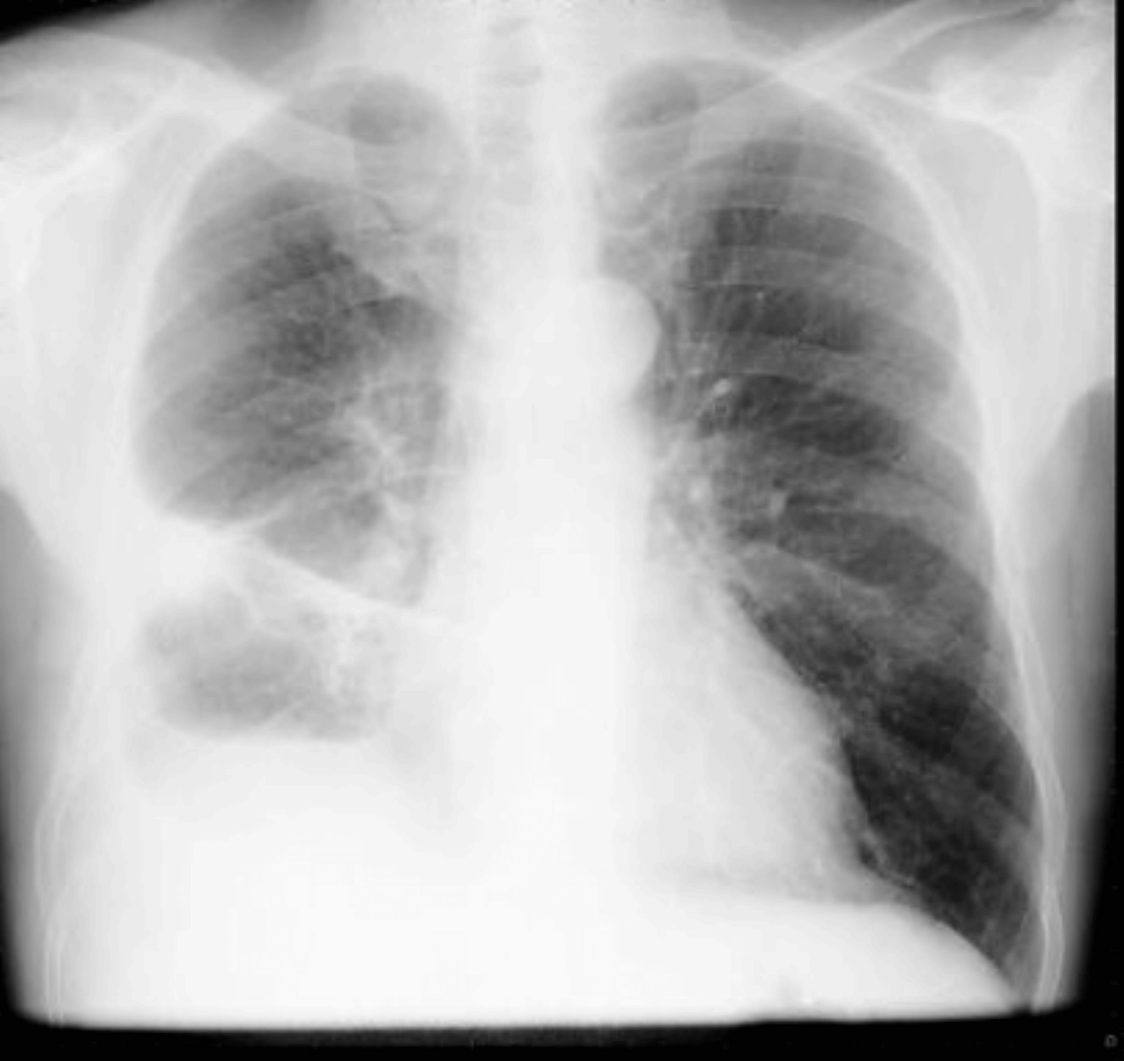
Chest X-ray on the 43rd day of hospitalization shows improvement after antibiotic therapy.
